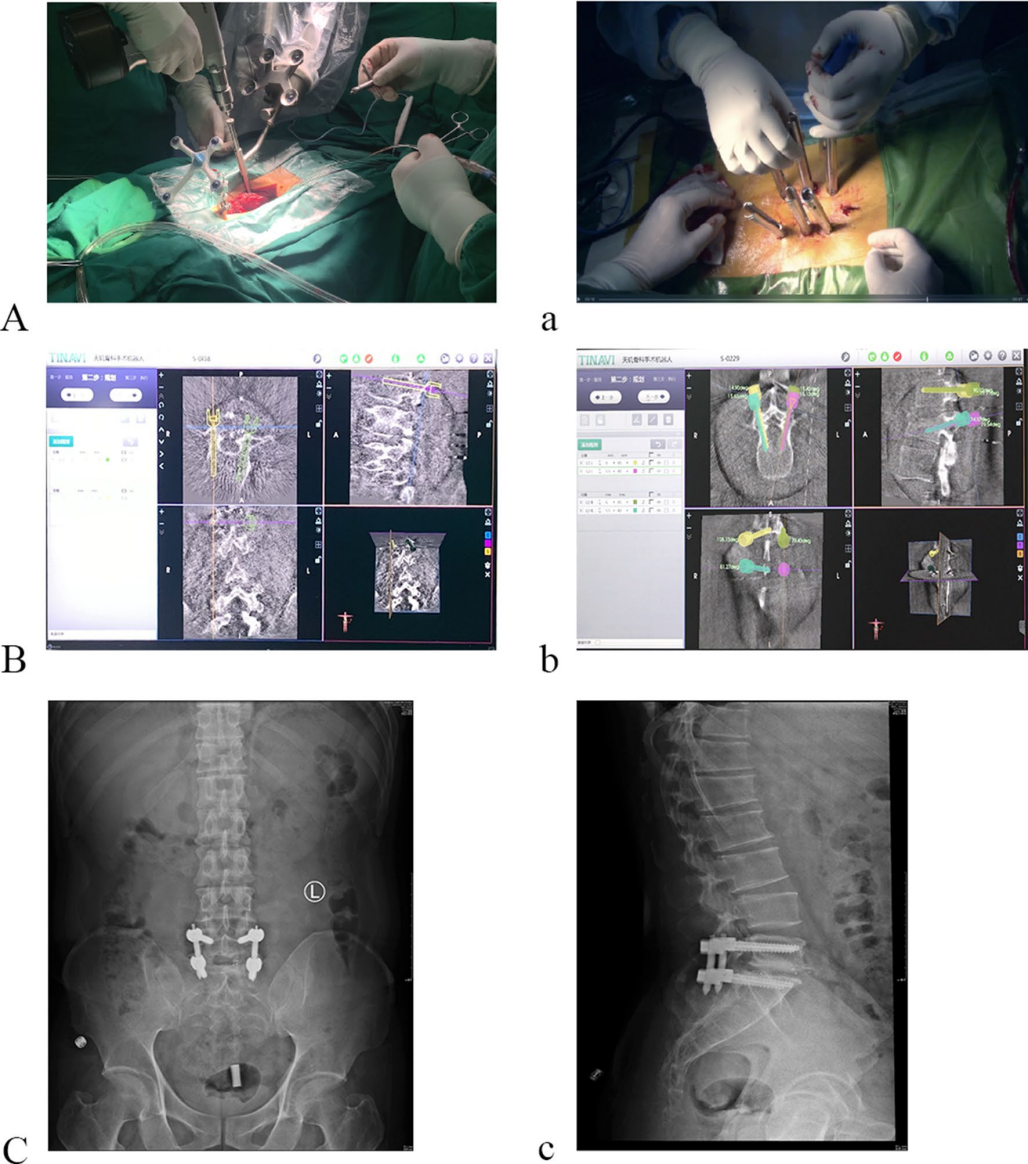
Clinical photographs, intraprocedural imaging and radiographs. A Surgery photo of RA-OS; a surgery photo of RA-MIS; B: Screw insertion design of cervical spine; b Screw insertion design of lumbar spine; C and c radiographs after lumbar surgery
